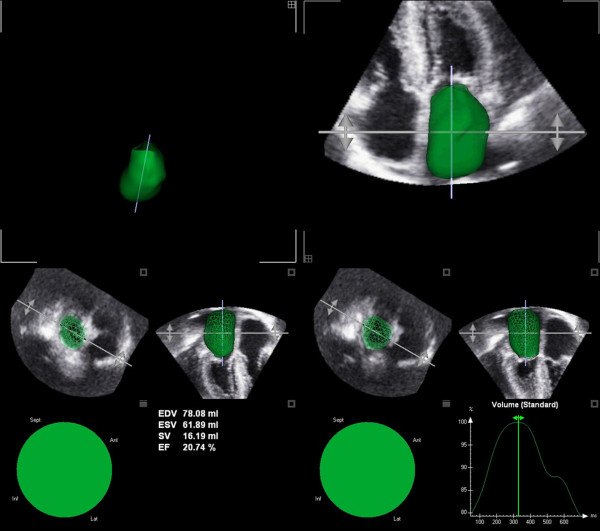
Three-dimensional echocardiographic LA reconstruction in a patient affected by cardiac amylodosis: LA enlargement and reduced LAEF.
